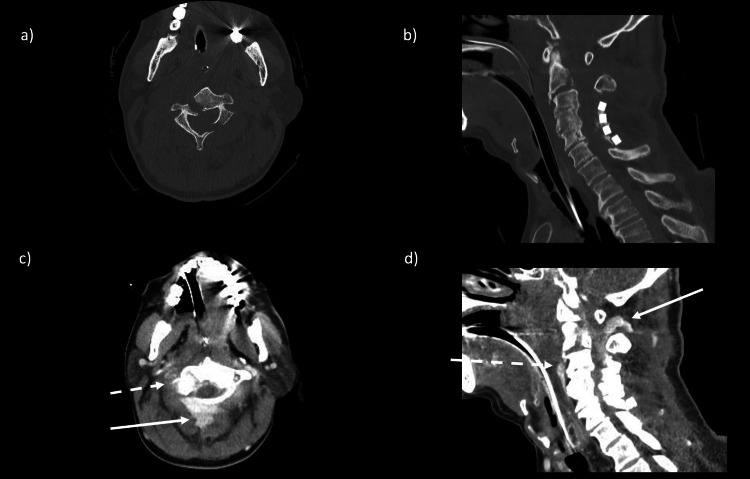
Plane CT neck in bone window algorithm, axial and sagittal views (a and b); enhanced CT neck in soft tissue algorithms, axial and sagittal views (c and d). a and b: Vertebral fracture of C2 and dislocation between C2 and C3 c and d: Marked extravascular leakage was seen on the anterior surface of the vertebral body (white dotted line), in the spinal canal of the cervical spine, and on the posterior surface of the vertebral body (white arrow).
